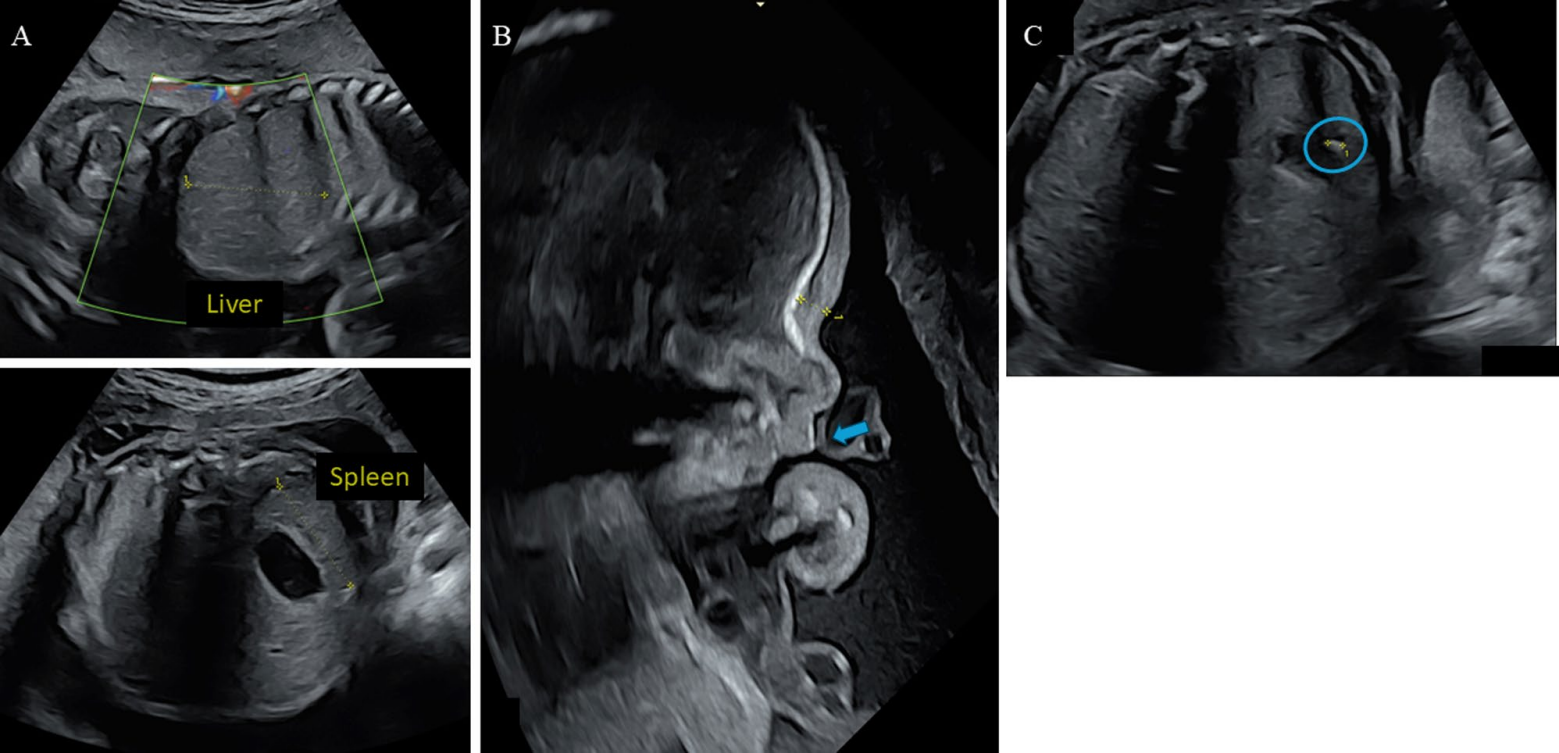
Morphological studies on Fetal Ultrasonic image at 28 GW. (A) Hepatosplenomegaly (measurements over + 2 SD). (B) Fetal profile with blue arrow pointing to the broad philtrum. (C) Peritoneal echogenic punctuation above the stomach (blue circle)
